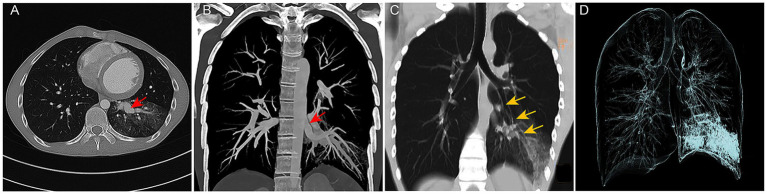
CT imaging findings of the patient’s chest. (A) On CT lung window settings, patchy hazy opacities are visible in the left lower lobe, with abnormally dilated and tortuous vessels forming a localized soft tissue nodule (indicated by red arrow). (B) A markedly enlarged anomalous artery originating from the distal thoracic aorta is observed coursing through the left lower lobe parenchyma (red arrows), accompanied by patchy consolidation and ground-glass opacities in the left lower lobe. (C,D) CT coronal reconstruction and 3D bronchial tree rendering demonstrate normal continuity between the left lower lobe bronchus and the native bronchial tree (yellow arrows).
